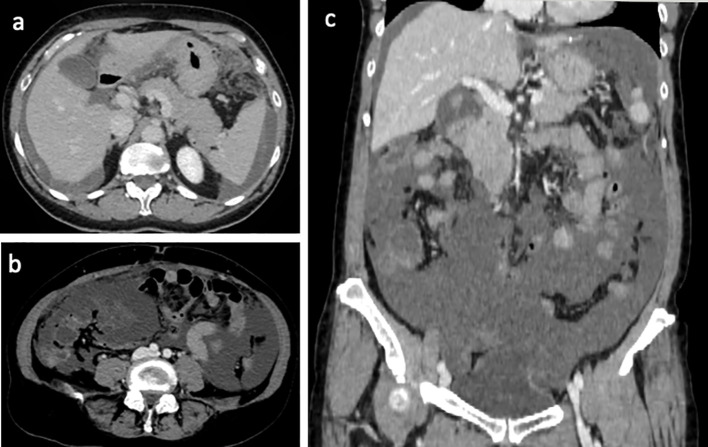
Axial (a, b) and coronal (c) portal CT scan showed ascites with liver scalloping and peritoneal implants.
